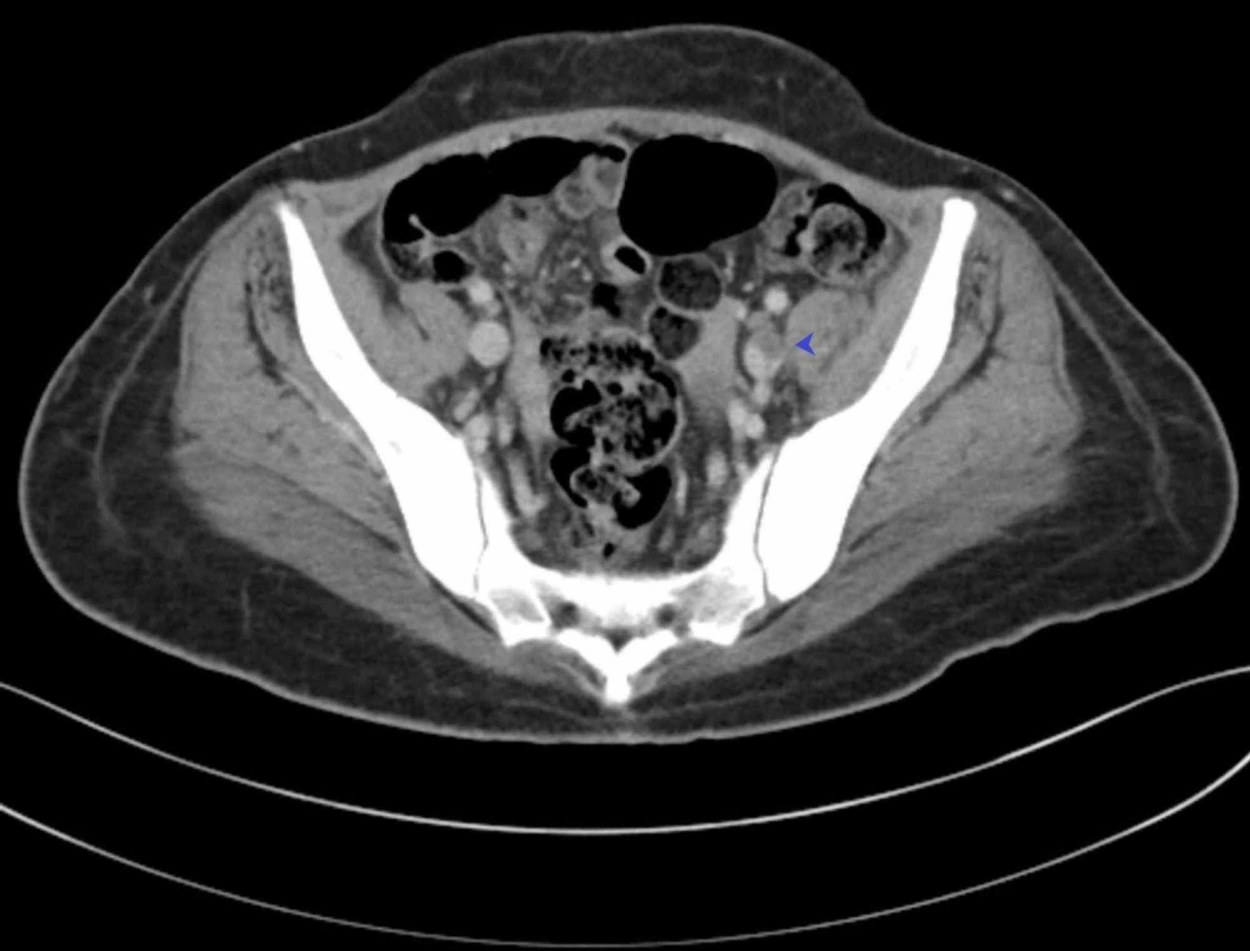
CT scan showing iliac vein thrombosis
